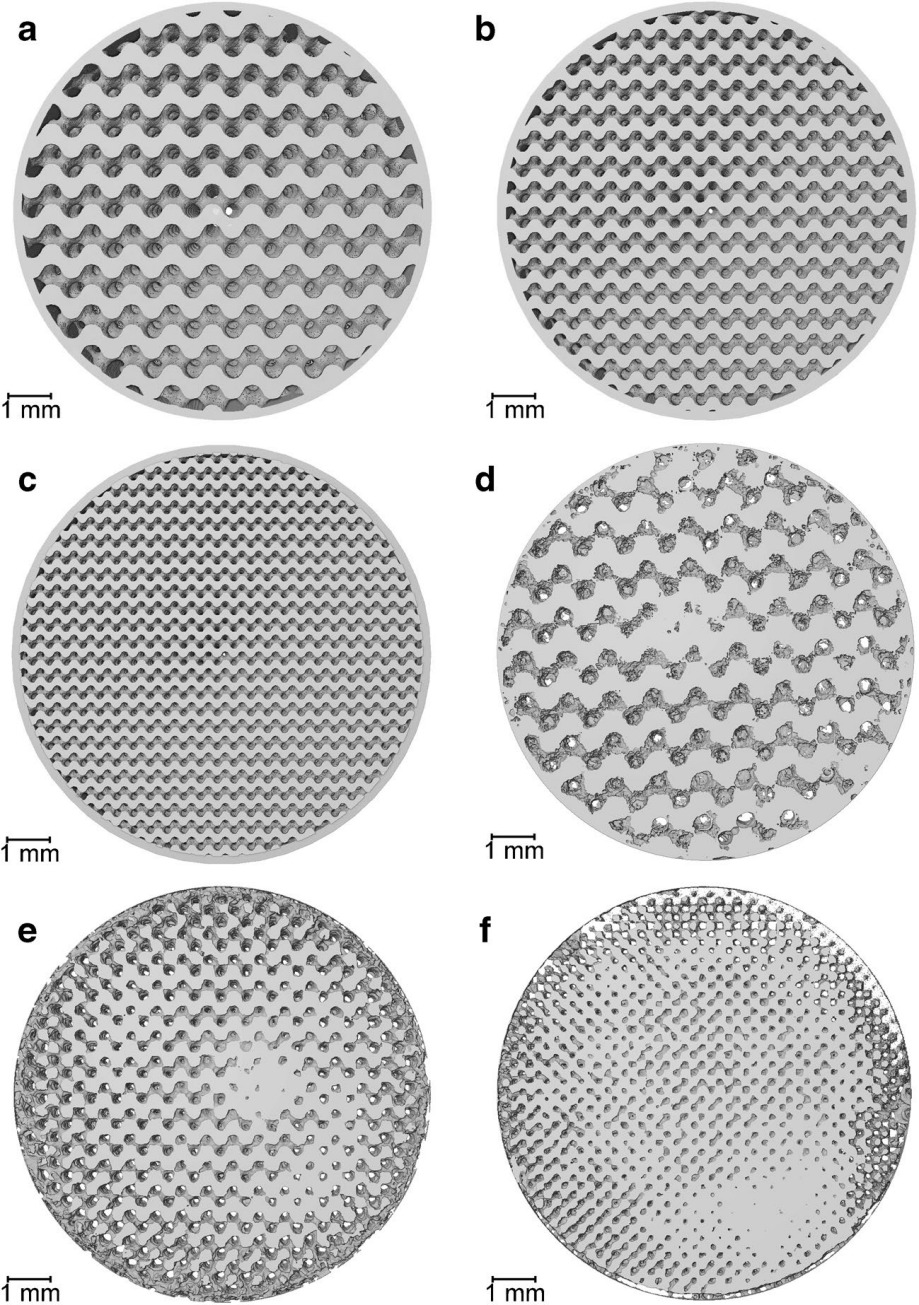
Whole gyroid design and imaging at a 5 µm pixel size. a Overhead CAD view with 500 µm feature sizes. b Overhead CAD view with 300 µm feature sizes. c Overhead CAD view with 200 µm feature sizes. d Overhead X-ray CT view of 3D-printed gyroid with 500 µm feature sizes. e Overhead X-ray CT view of 3D-printed gyroid with 300 µm feature sizes. f overhead X-ray CT view of 3D-printed gyroid with 200 µm feature sizes
